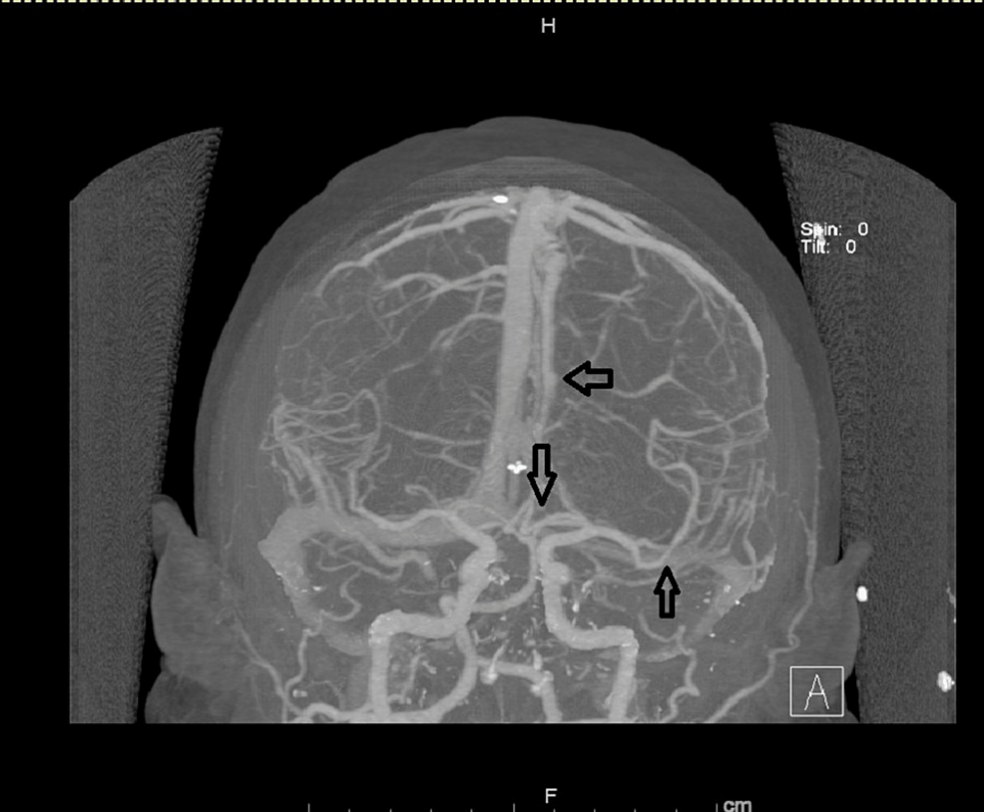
A follow-up computed tomography angiography (CTA) of the brain CTA brain showing relative improvement of prior multifocal stenosis affecting left M2, left A1, and A3 (arrows).
